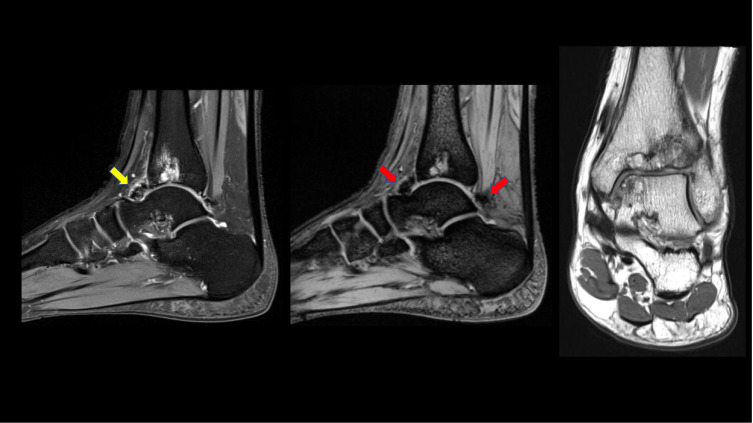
MRI of the ankle joint: sagittal T2WI, sagittal gradient sequence and coronal PDWI, show intra-articular synovial low signal intensity (yellow arrow) demonstrating blooming artifact on the gradient sequence (red arrows) in the anterior and posterior recess of the ankle joint consistent with blood degradation products related to repeated episodes of hemarthrosis. Again, secondary osteoarthritic changes are noted with multiple cystic changes/geodes with surrounding marrow oedema. Findings are consistent with siderotic chronic synovitis.
